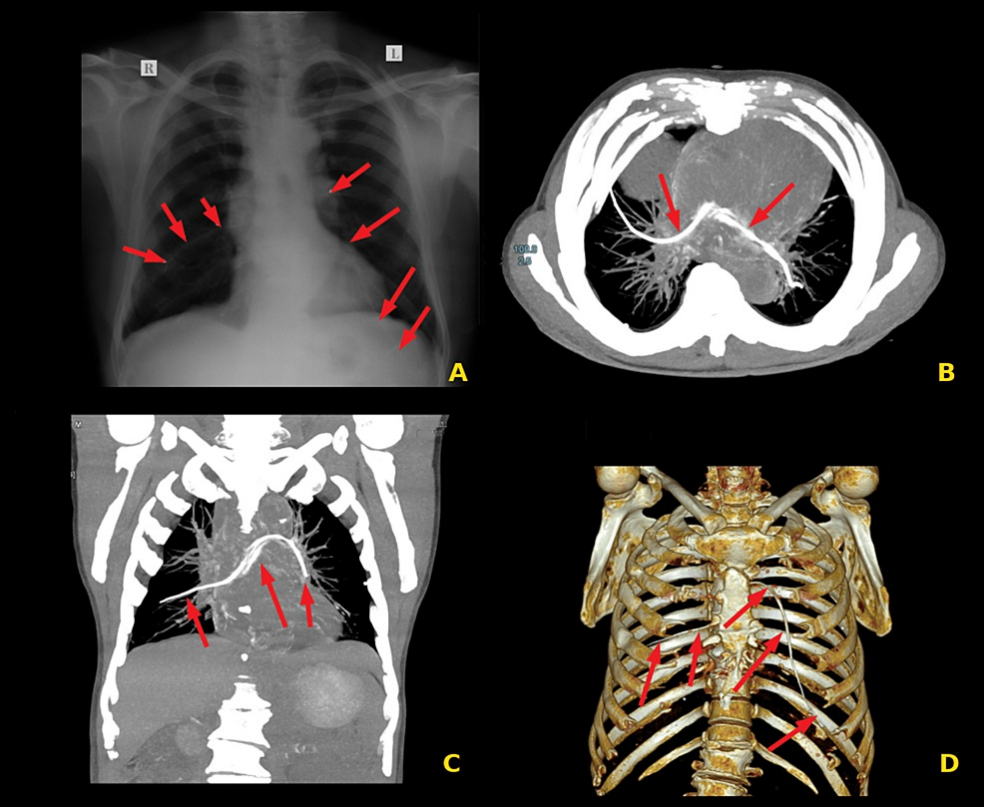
(A) Chest X-ray showing broken PICC line fragments (arrows) in bilateral lungs extending into peripheral pulmonary vasculature; (B) axial CT image revealing the migrating PICC in the right pulmonary artery's right middle lobe segmental branch (arrows). It is also seen extending into the left lower lobe segmental branch of the left pulmonary artery (arrows). The proximal end of the broken PICC was noted in the region of the main pulmonary artery bifurcation; (C) coronal CT image revealing the migrating PICC in the right middle lobe segmental branch of the right pulmonary artery (arrows). It is also seen extending into the left pulmonary artery (arrows); (D) 3D reconstructed CT images revealing the migrated PICC extending into the segmental branches of bilateral pulmonary arteries (arrows) PICC, peripherally inserted central catheter
